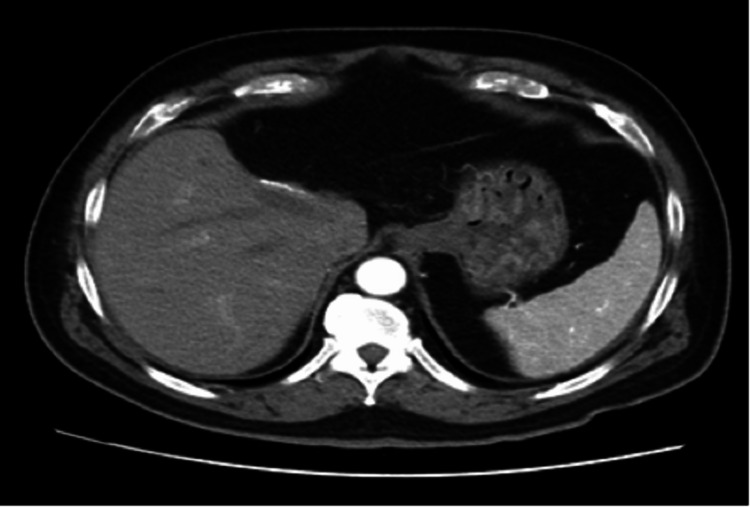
Contrast-enhanced CT showing metallic suturing at the resection site with internal non-visualization of a previously identified left hepatic lobe lesion.
